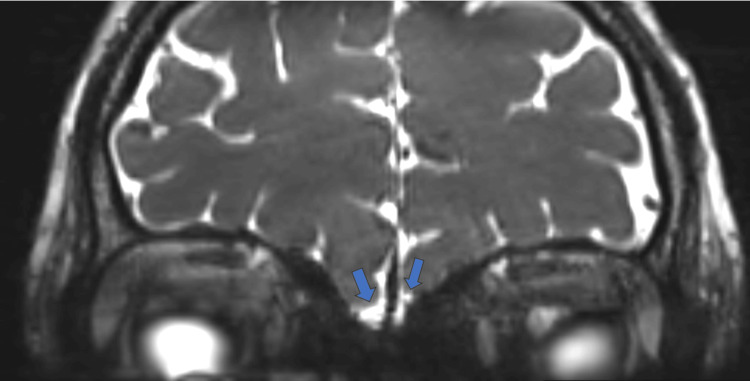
CISS (constructive interference in steady state) MRI sequence showing absent olfactory bulbs (blue arrows).
